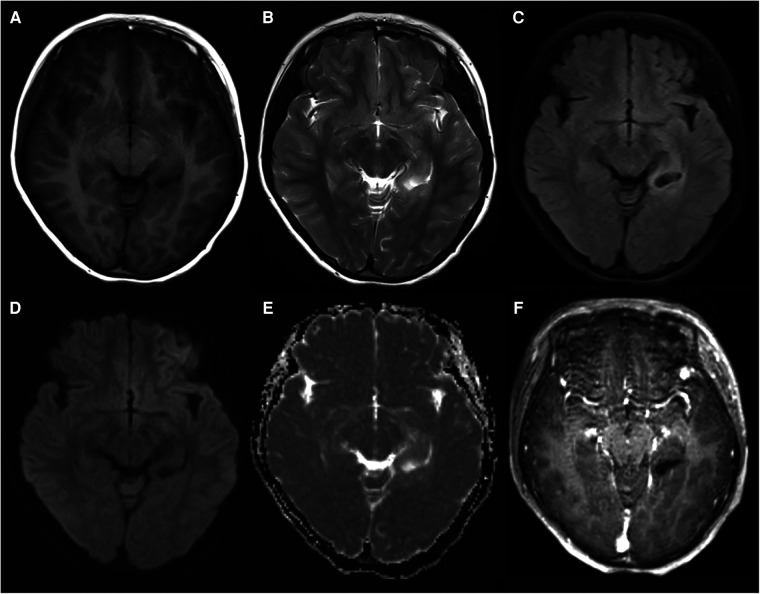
Pediatric-type diffuse low-grade glioma, MAPK pathway-altered in a 10-year-old girl. The tumor is located in the left hippocampus and shows hypointense on T_1_WI (A) and hyperintense on T_2_WI (B) and FLAIR (C). Diffusion is not restricted (D,E). No enhancement is observed (F).
